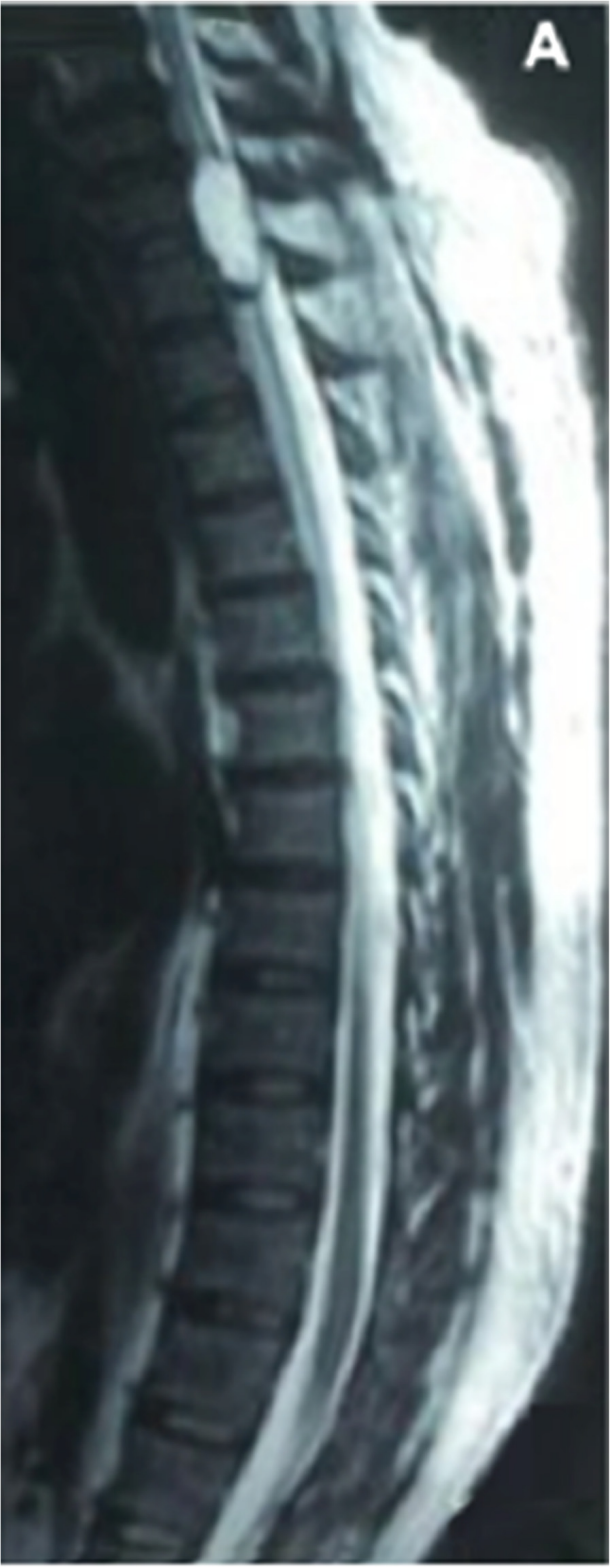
Thoracic MRI. Sagital T2 MR image of the thoracic region demonstrating round intramedullary mass at C7 to T1 level meassuring 33 × 12 mm.
